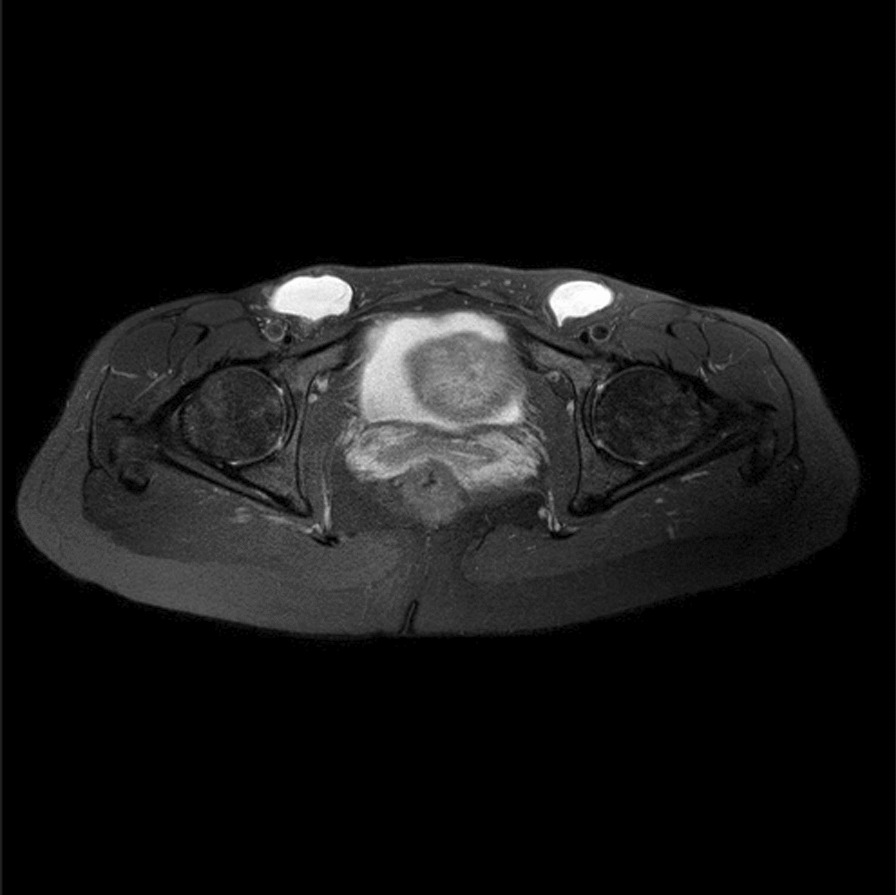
Preoperative MRI, T_2_ weighted, showing bilateral cystic protrusions of the groin
